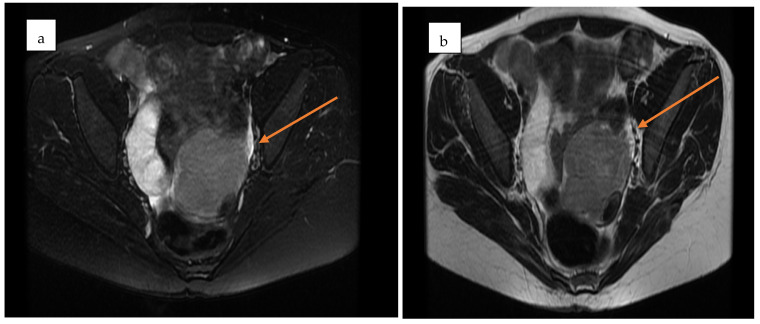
Axial pelvic MRI of the adnexal mass in the pouch of Douglas. (a) Pre-contrast T2-weighted image showing a well-encapsulated, septated mass with mixed cystic–solid components (yellow arrow). (b) Post-contrast image demonstrating enhancement of the solid nodular component, consistent with vascularization (yellow arrow).
